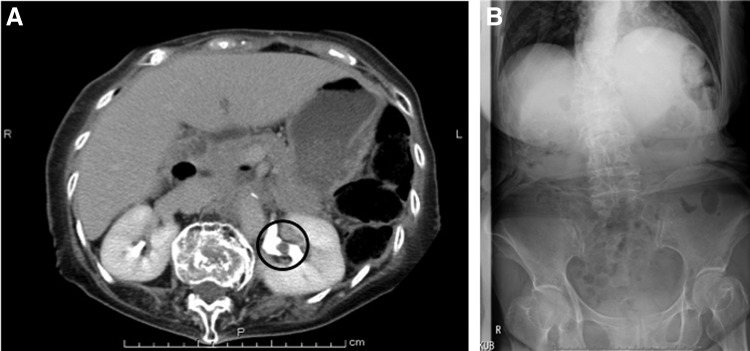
(A) CT scans showed a slightly enhanced mass in the left renal pelvis. (B) Radiography showed curvature of the spine and well-creased abdomen. Urothelial carcinoma (indexed in the *black circle*) was suspected.
